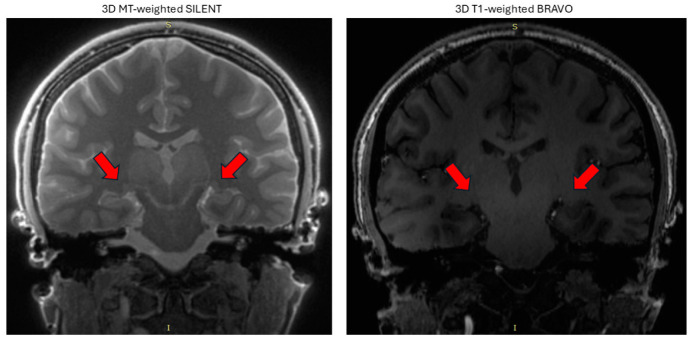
Comparison of lateral geniculate nucleus (red arrows) visibility in two sequences. On the left side is 3D MT-weighted SILENT; on the right side is 3D T1-weighted BRAVO. Images acquired at the Ecotech Complex (Lublin, Poland).
